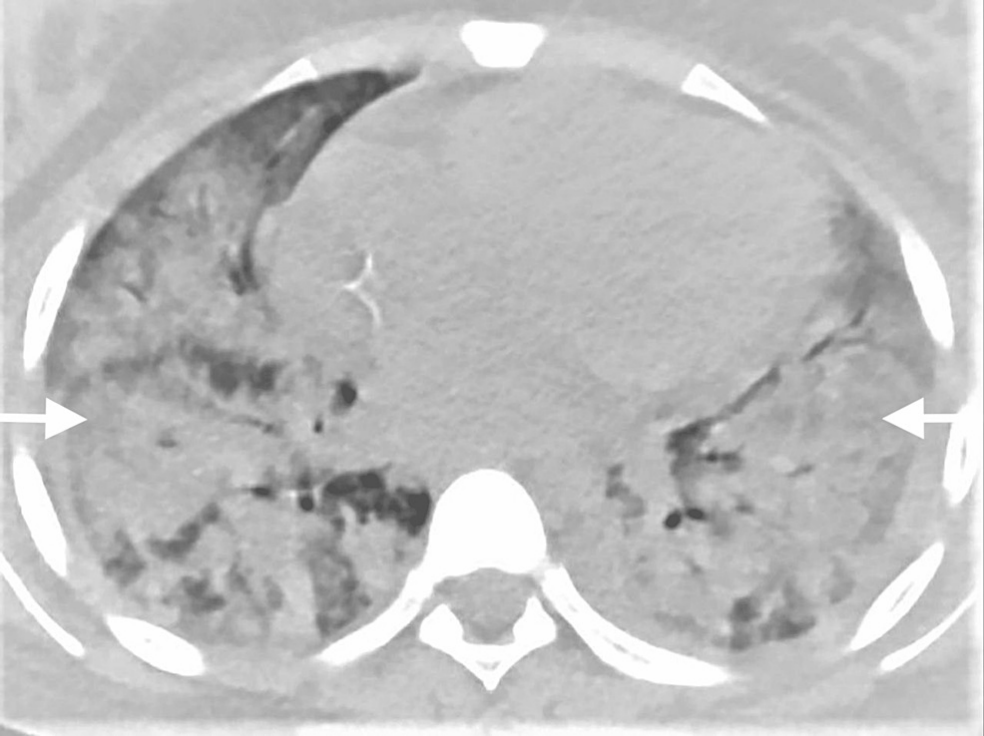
High-HRCT before treatment showing bilateral diffuse alveolar hemorrhagic infiltration. HRCT: resolution computed tomography
